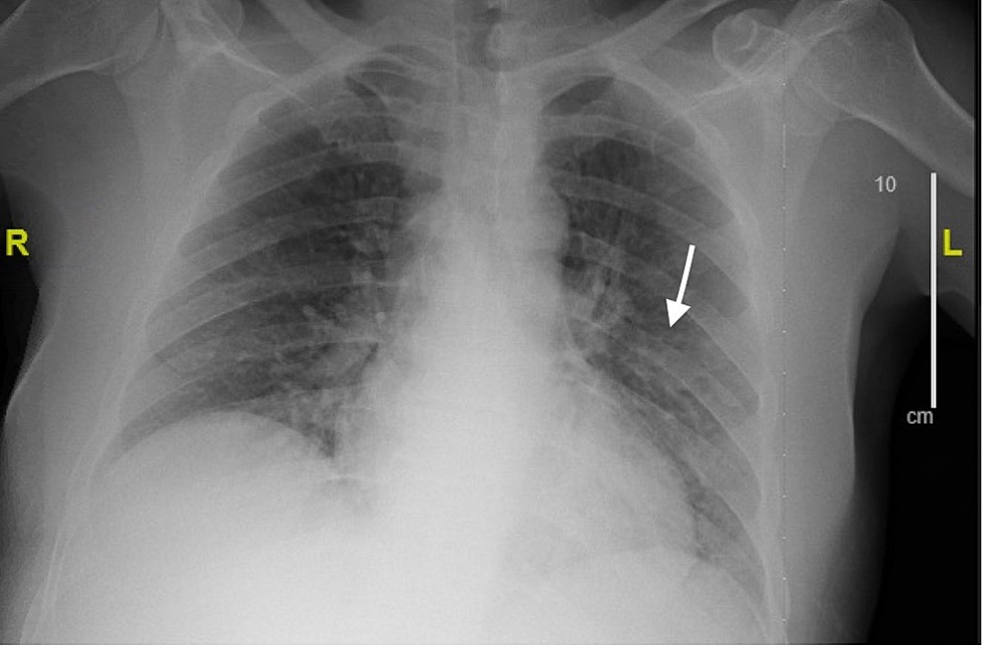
Chest X-ray significant of a left basilar opacity (arrow), most likely to represent consolidation, concerning for pneumonia
